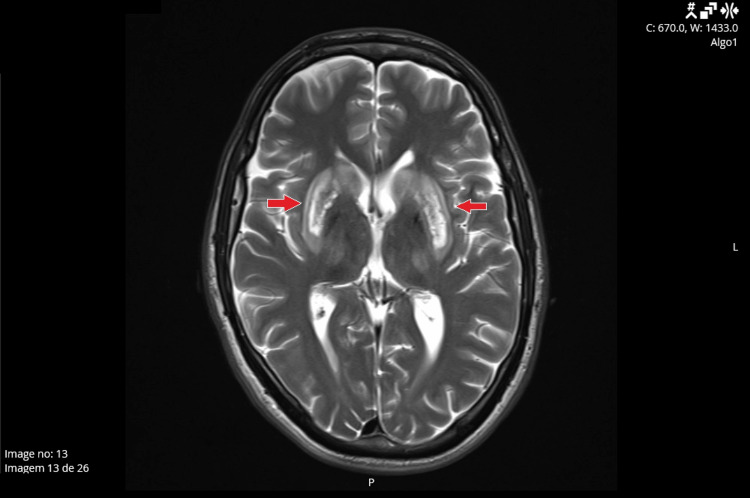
Brain MRI. Axial cuts of the T2 sequence of the patient’s brain MRI with contrast in January 2024 demonstrate a hypersignal and edema bilaterally in the putamen and caudate nuclei, along with a hypersignal on the globus pallidus, translating likely to copper deposits. The arrows demonstrate the “bright claustrum sign” in this patient’s image, which is attributed to a hypersignal of the claustrum.
